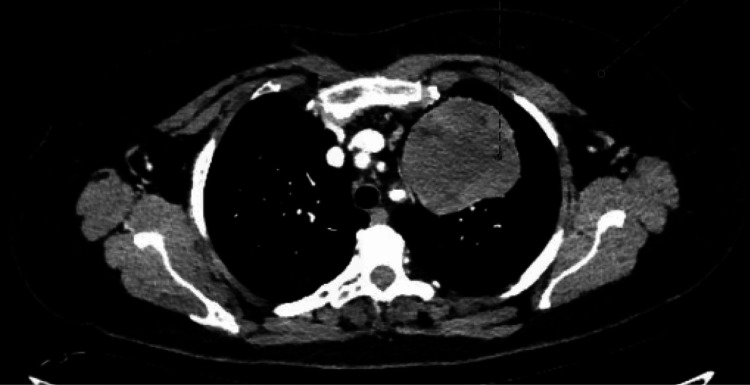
Axial view contrast-enhanced CT thorax showing a well-circumscribed anterior mediastinal hamartoma with heterogeneous attenuation, containing focal areas of macroscopic fat, causing mild mass effect on adjacent mediastinal structures without invasion.
